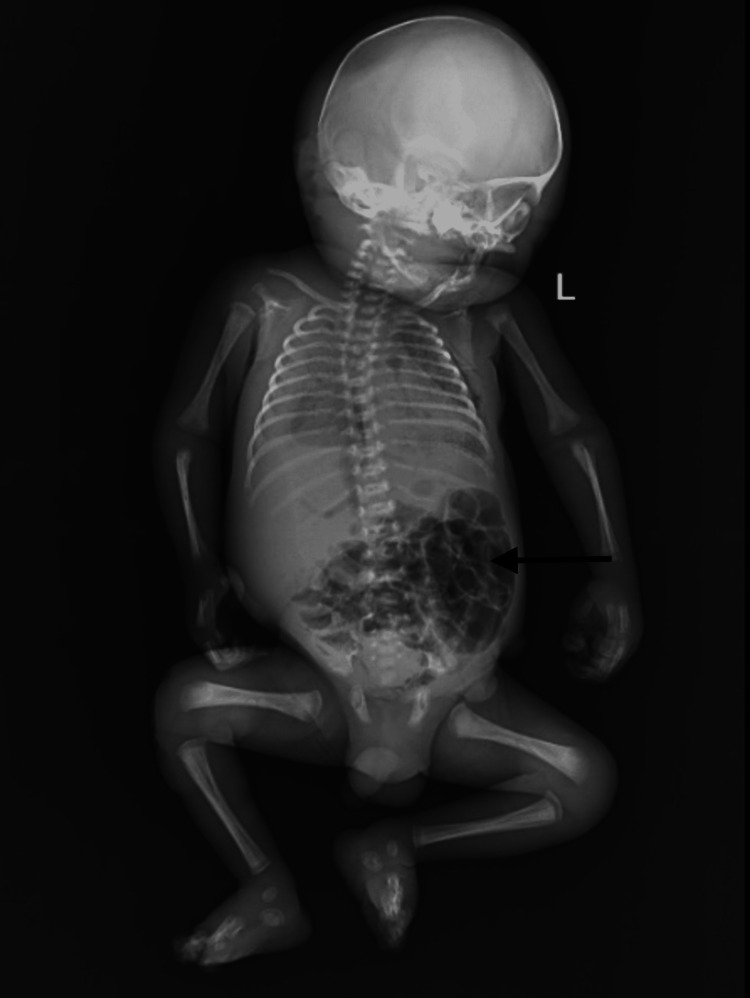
Fetal X-ray depicting absent bowel gas shadows (arrow) in the rectum consistent with an imperforate anus
